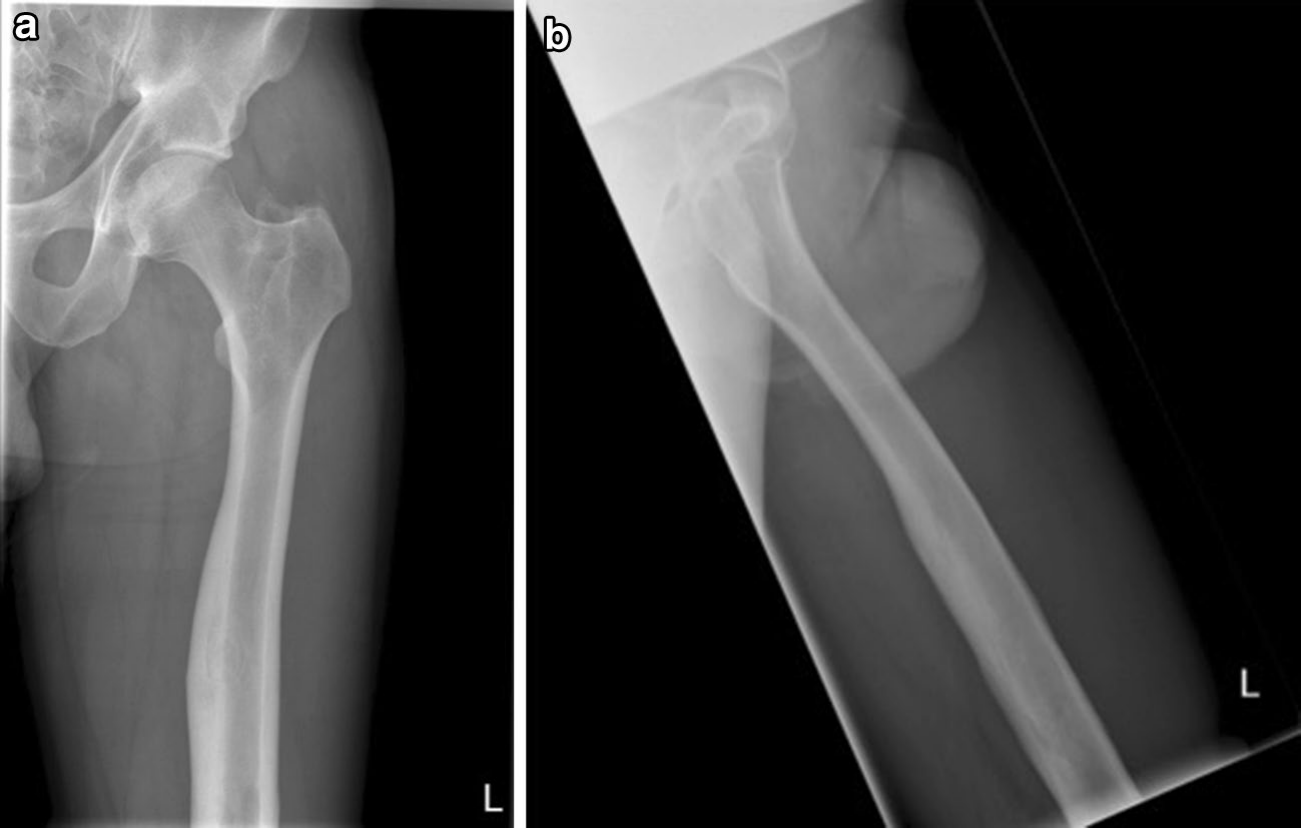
a Anteroposterior and b lateral radiographs of the *left* femur 12 months after the removal of the rod
